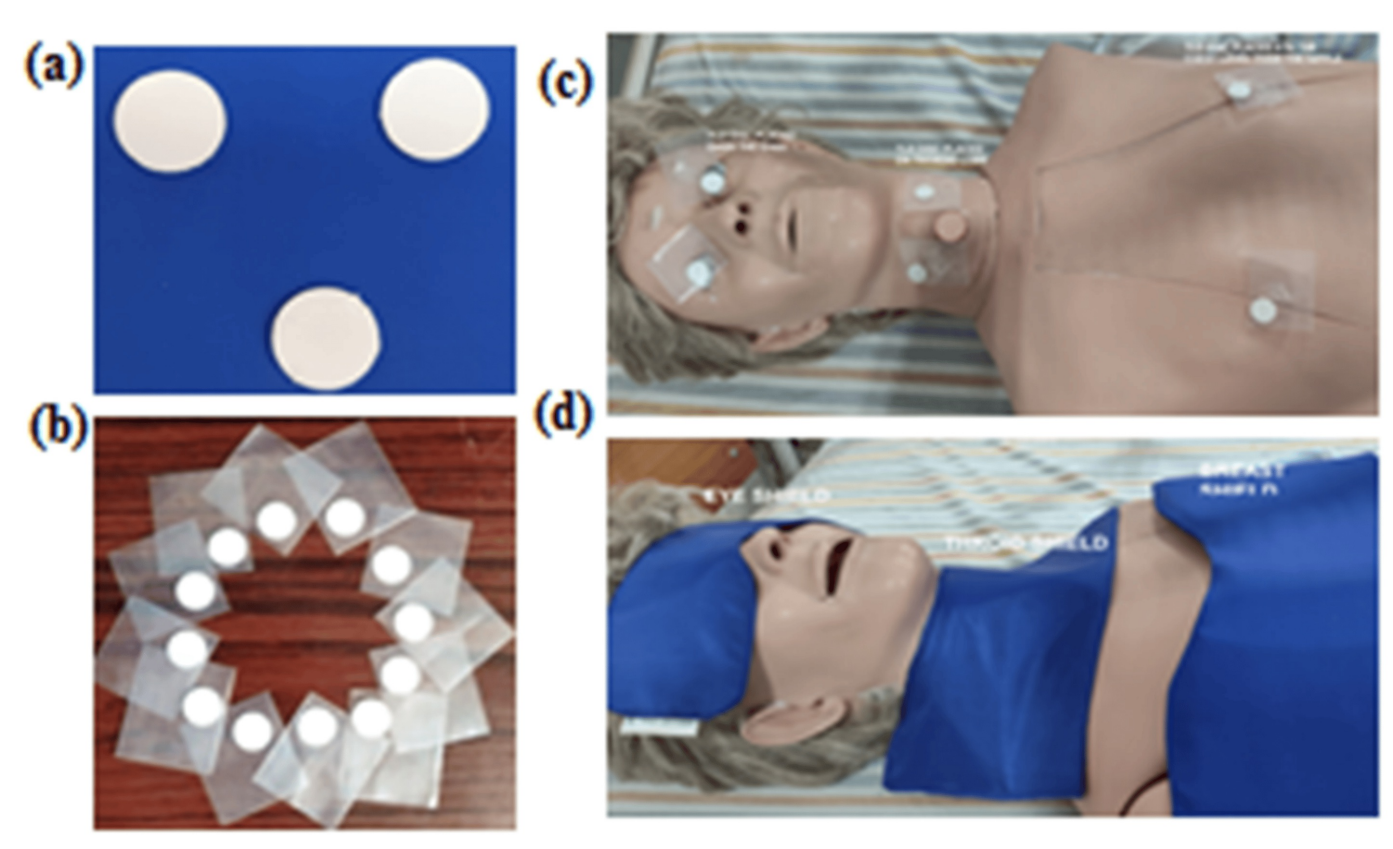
Representative images used in the study (a, b) CaSO₄:Dy Teflon TLD discs. (c) Placement of TLD discs on the eyes, thyroid, and breasts of participants undergoing plain abdominal CT scans. (d) Lead shielding devices are designed for the eyes, thyroid, and breasts. These images demonstrate the methodology for radiation dose measurement and shielding application. TLD: thermoluminescence dosimeter, CT: computed tomography
